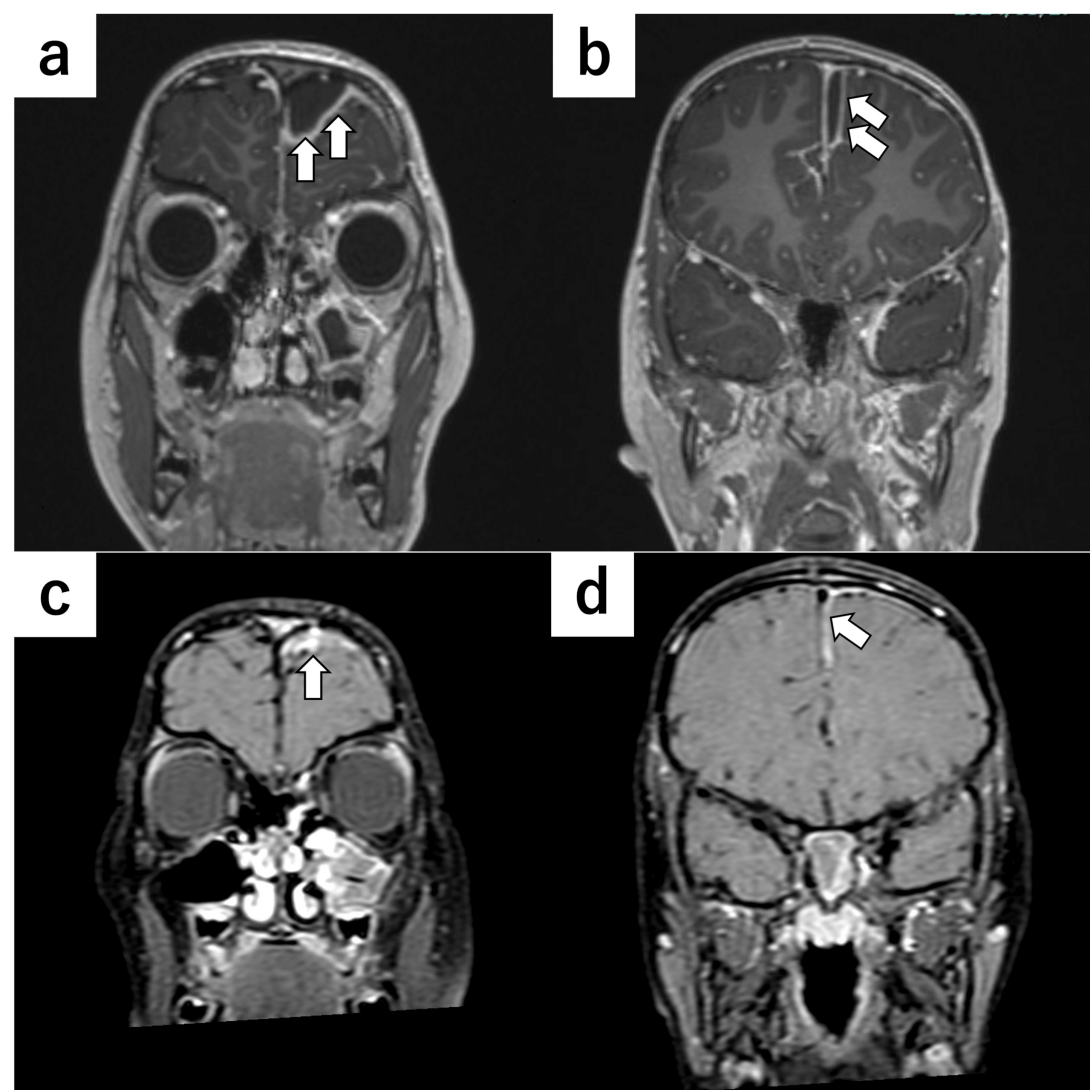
Coronal MRI of the patient with parasagittal frontal epidural abscess and interhemispheric subdural empyema Preoperative gadolinium-enhanced T1-weighted images (a: epidural abscess, b: subdural empyema) show rim-enhancing low-signal-intensity lesions, while postoperative fat-suppressed gadolinium-enhanced T1-weighted images obtained 19 days after surgery (c: epidural abscess, d: subdural empyema) demonstrate a marked reduction of both lesions. MRI: magnetic resonance imaging
